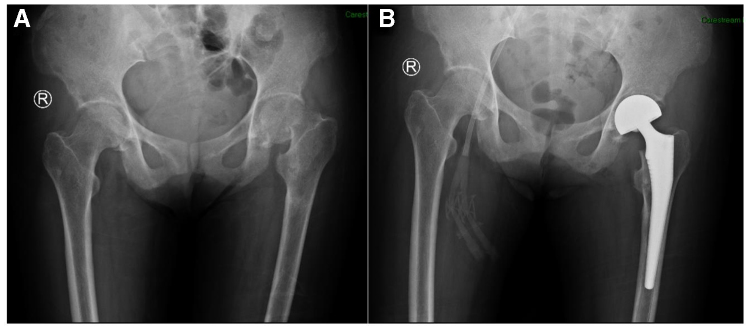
Preoperative (A) and postoperative (B) radiographs of the hip. The preoperative pelvic radiograph shows a displaced subcapital femoral neck fracture, and the postoperative image demonstrates a hemiarthroplasty.
